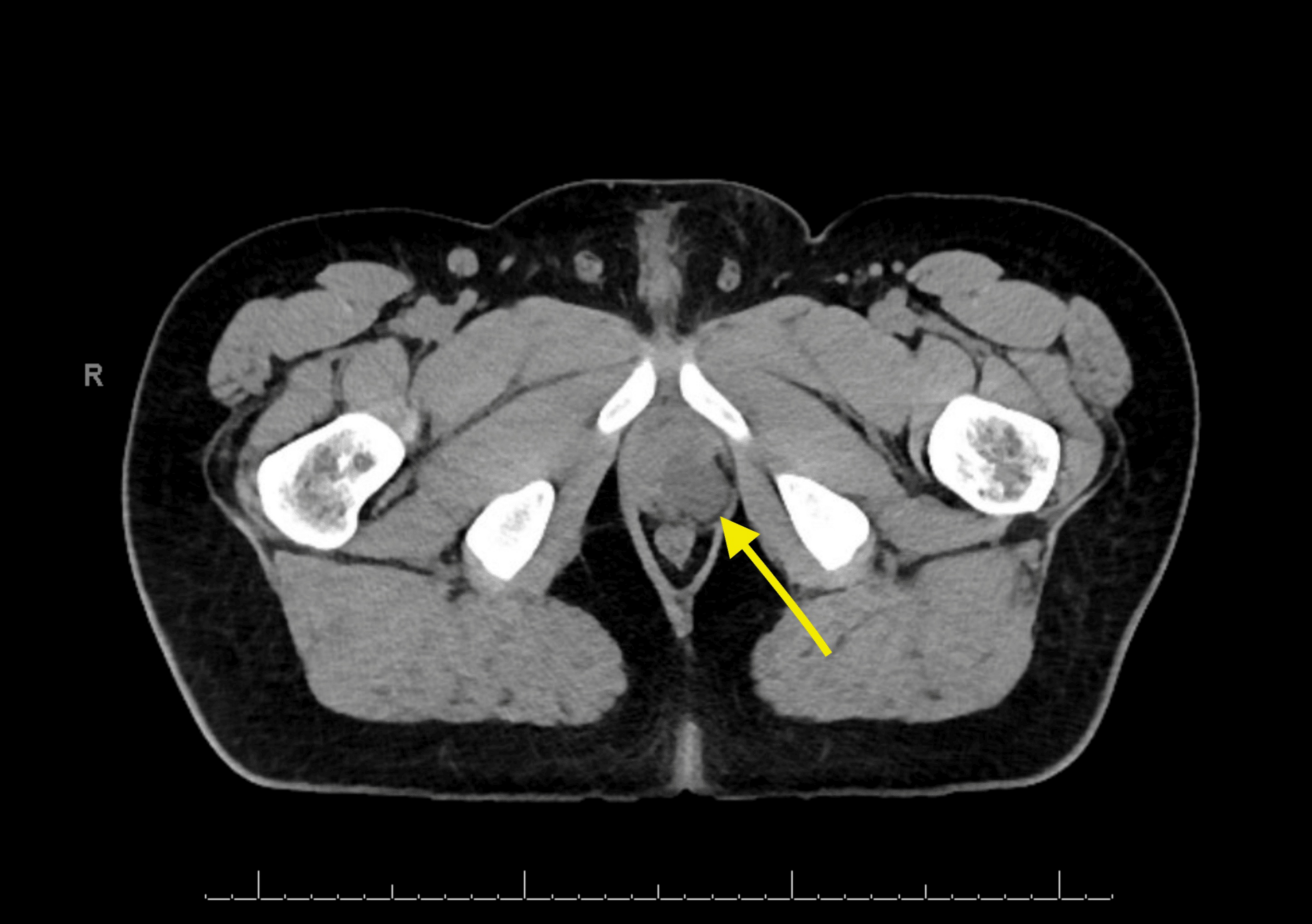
CT of the pelvis with the yellow arrow demonstrating dilated seminal vesicle.
